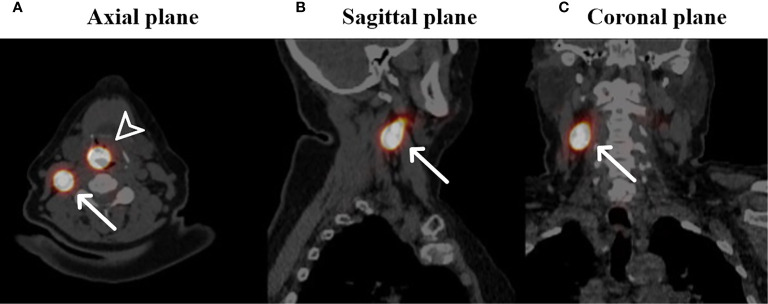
Fused SPECT-CT images of a patient with a cT1N0 squamous cell carcinoma located on the laryngeal side of the epiglottis who underwent SN identification (own unpublished data). Arrowhead: primary injection site of ^99m^Tc radioisotope, white arrows: identified SN in level II on the right side in the axial plane (A), sagittal plane (B) and coronal plane (C).
